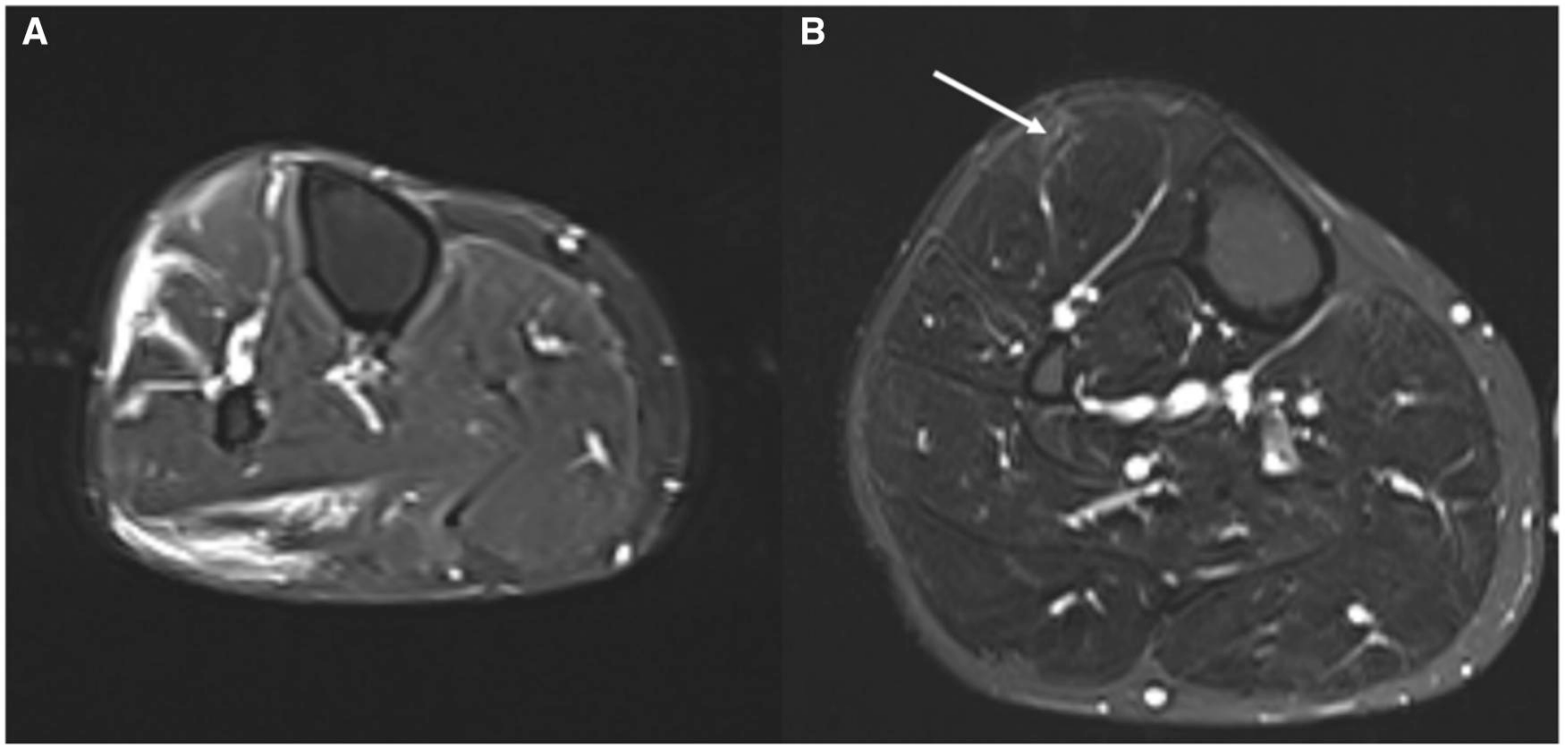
MRI of the lower legs. (A) At the time of initial presentation, prior to any treatment, showing diffuse myo-oedematous and myofascial changes. (B) Following treatment with steroid, infliximab and azathioprine 6 months later. The white arrow shows a tiny focus of residual oedematous change anterior to the tibialis anterior tendon on the right and the patient continued to report ongoing pain here
